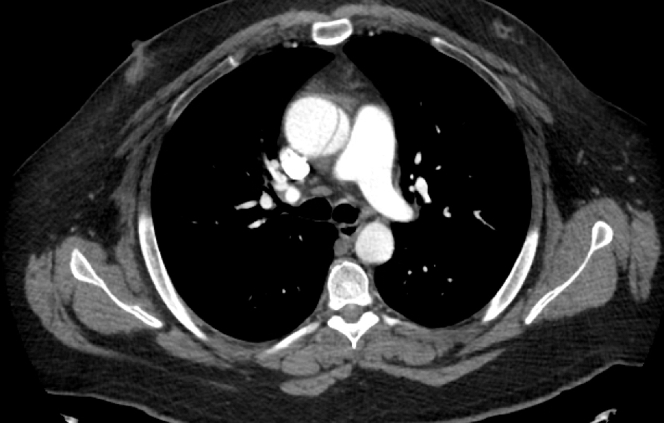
CT Angiography Chest, Abdomen, and Pelvis: Axial View CT angiography demonstrating ascending aortic dissecting aneurysm Stanford type A with visualization of the intimal flap, a hallmark sign. There is also involvement of the sinus of Valsalva where the aorta measures 7 cm, cardiomegaly with left ventricular dilatation, and dissection involvement of the right brachiocephalic artery. Contrast density is symmetric within the true and false lumen. CT = computed tomography.
